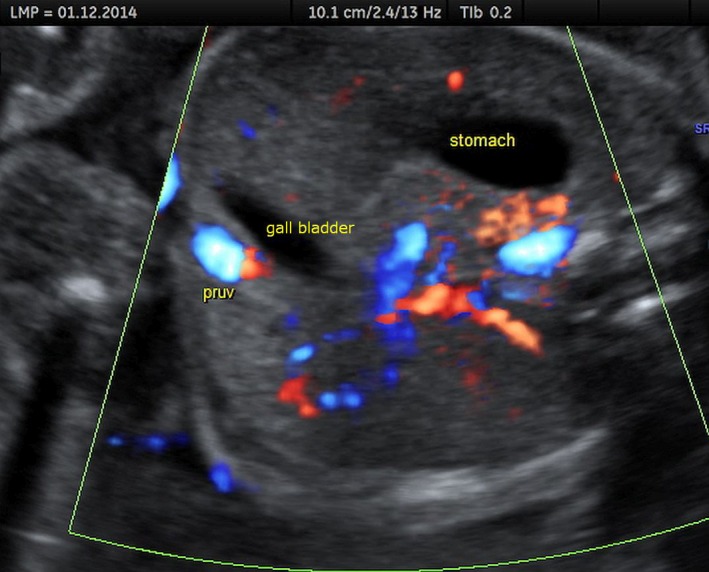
Persistent right umbilical vein (PRUV) visualised in a cross‐section of the fetal abdomen (colour Doppler). Gallbladder is situated medial to the UV.
